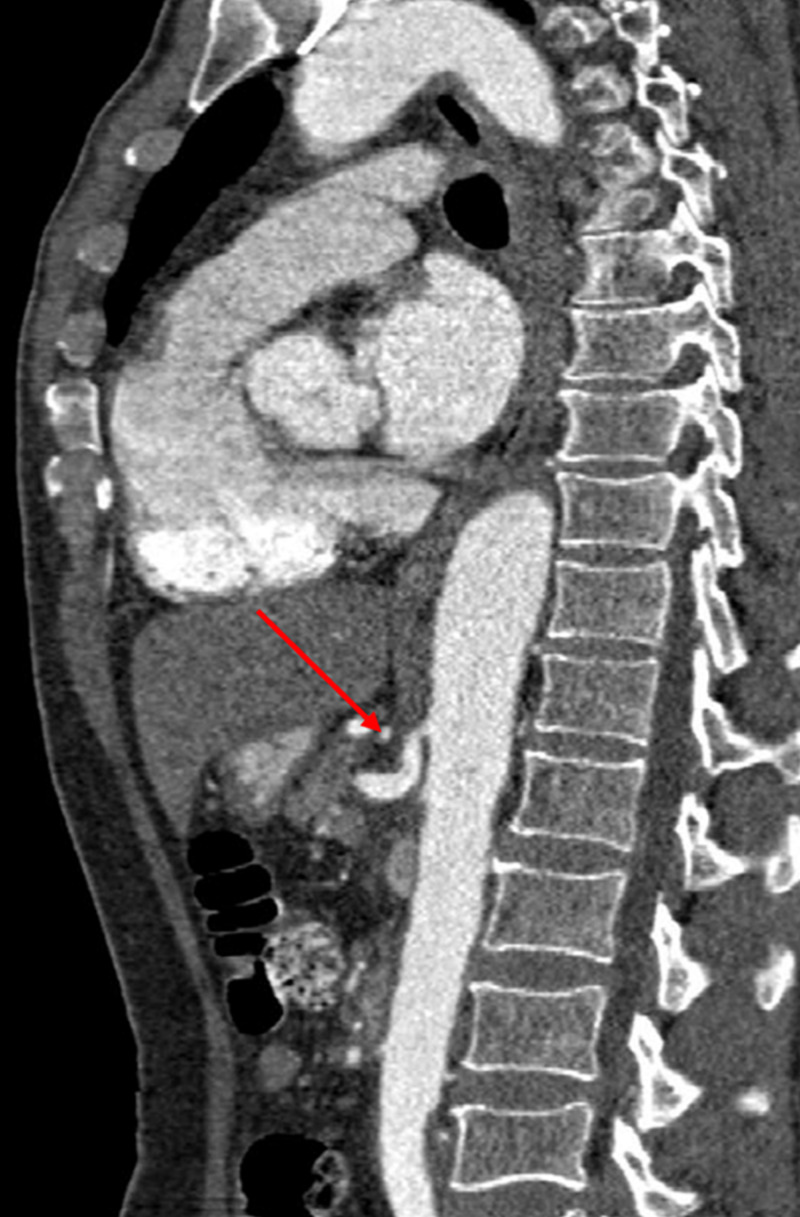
Sagittal reformatted CT aortogram image at the level of CA origin. Note the dissection in the proximal segment of CA (red arrow). CA, celiac artery
